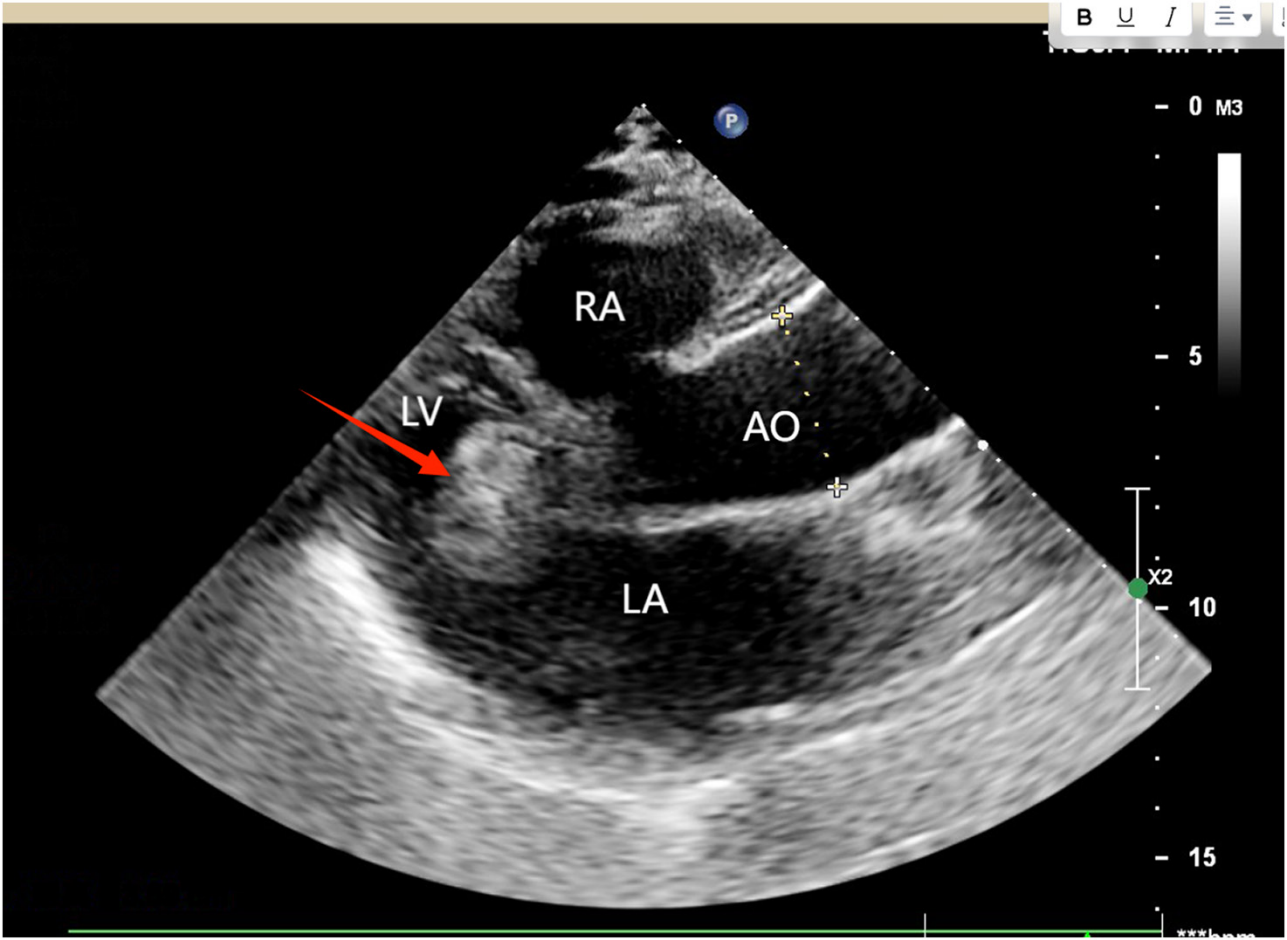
Echocardiography indicates occlusion device displacement (The occluder is located in the left ventricle).
